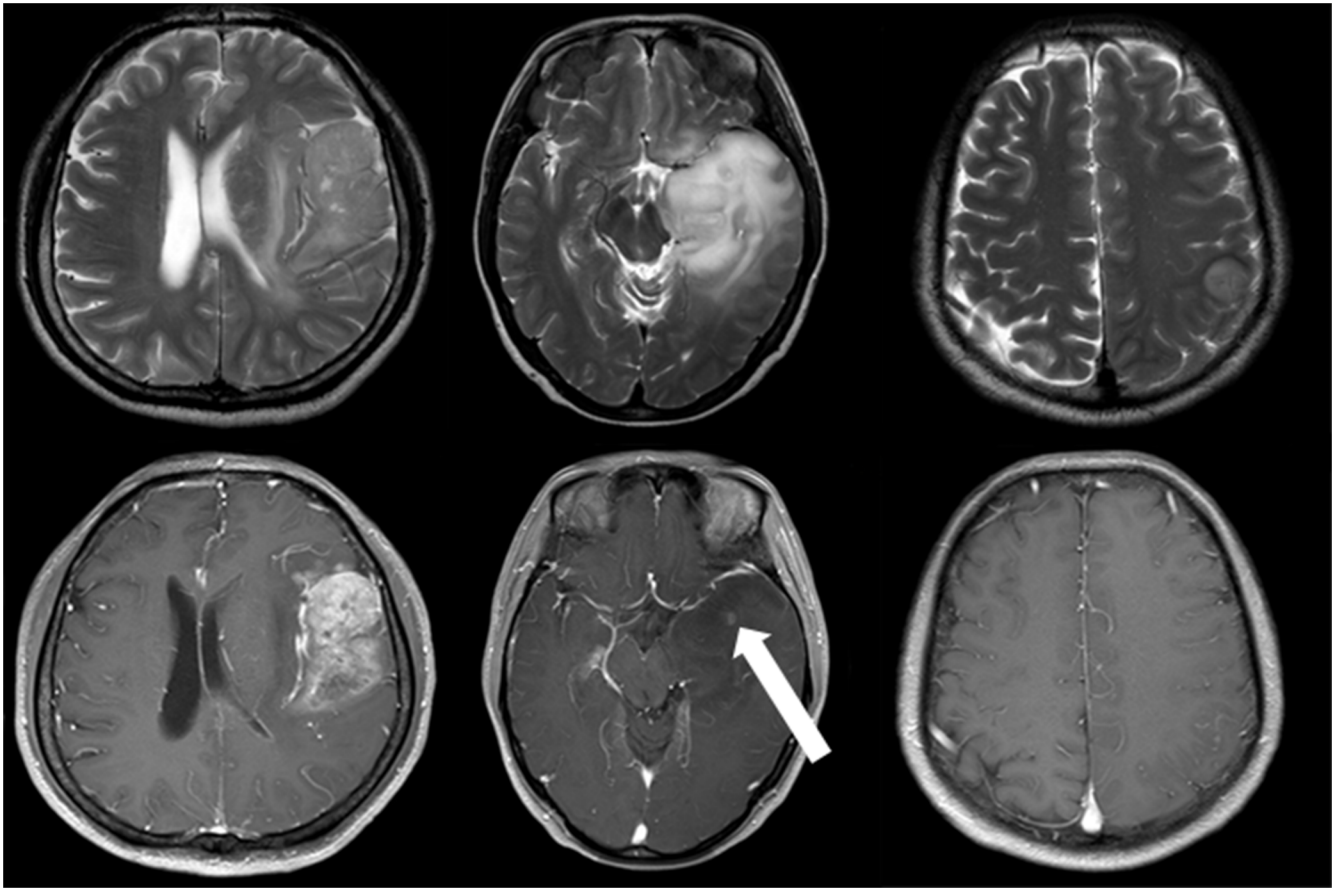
Examples of different enhancement extent. The left column is a case of glioblastoma with apparent enhancement, the middle a case of high-grade glioma with slight enhancement (white arrow) and the right a low-grade glioma with negative enhancement. Upper row axial T2-weighted images. Lower row axial T1W+C images.
